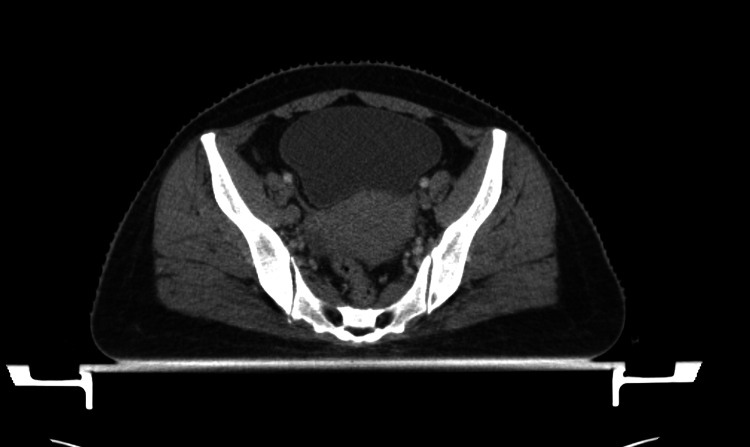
Baseline CECT of the whole abdomen (axial section) displaying sub-centimetric lymph nodes along with the main tumor. CECT: contrast-enhanced computed tomography
